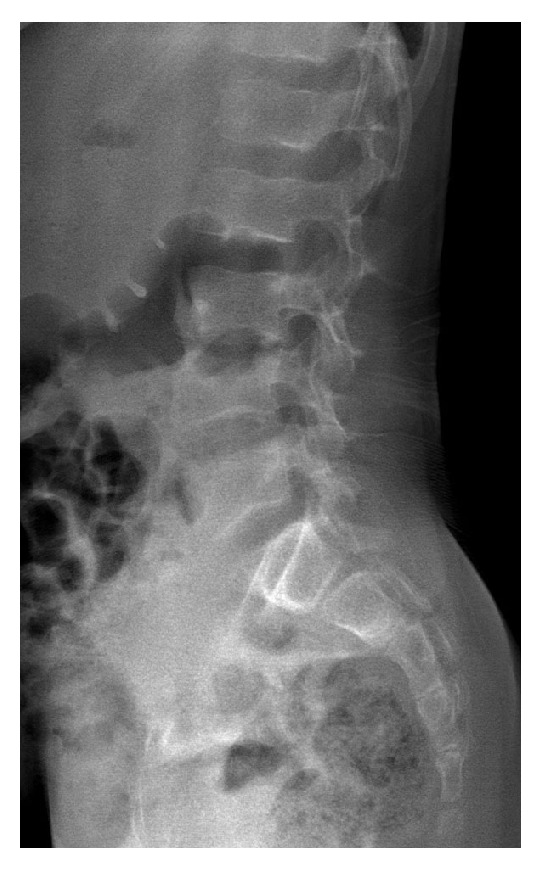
Lateral radiograph of the lumbar spine 2 months after the surgery shows complete collapse of the L3-L4 disk space with marked sclerosis, irregularities, and erosion of the inferior endplate of L3 and superior endplate of L4.
